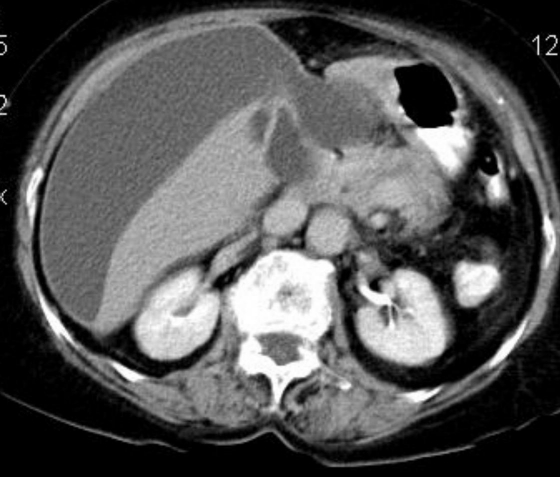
Contrast enhanced computed tomography scan of the abdomen revealed abdomen revealed gall bladder perforation with perihepatic collection.
